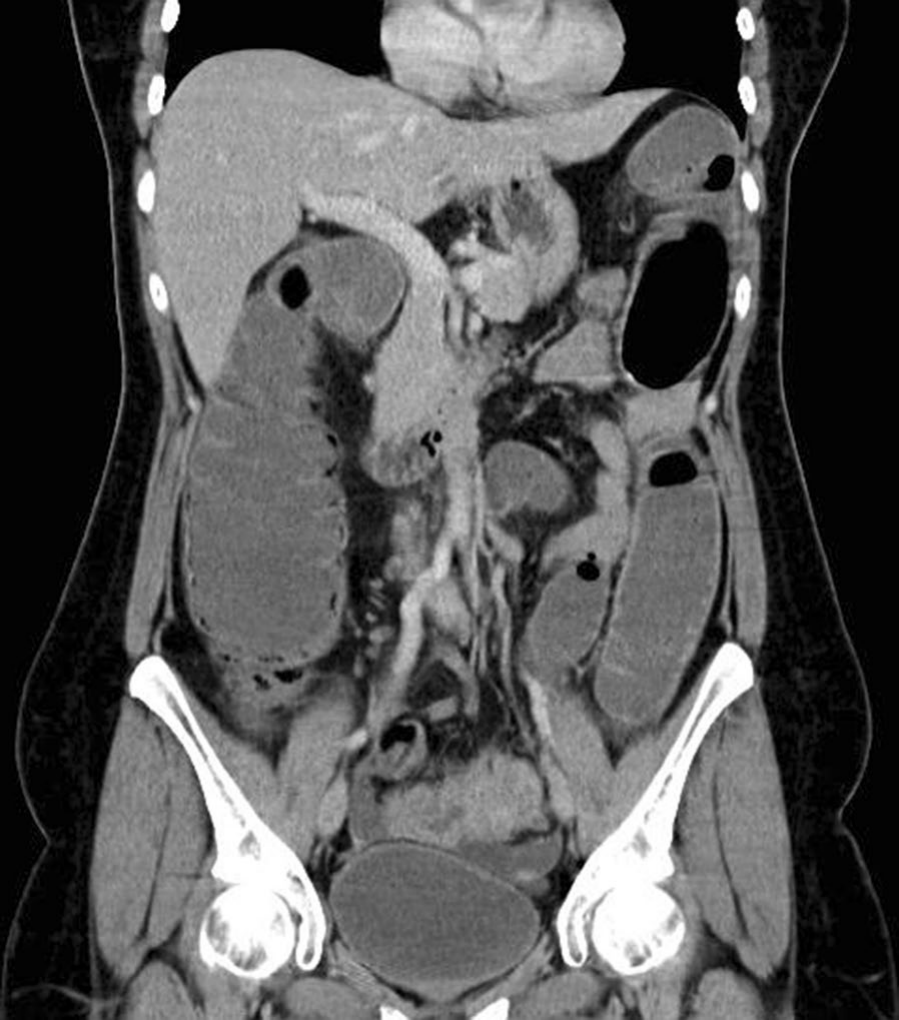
CT images demonstrating thick sigmoid colon with large bowel obstruction and left ovarian cyst
